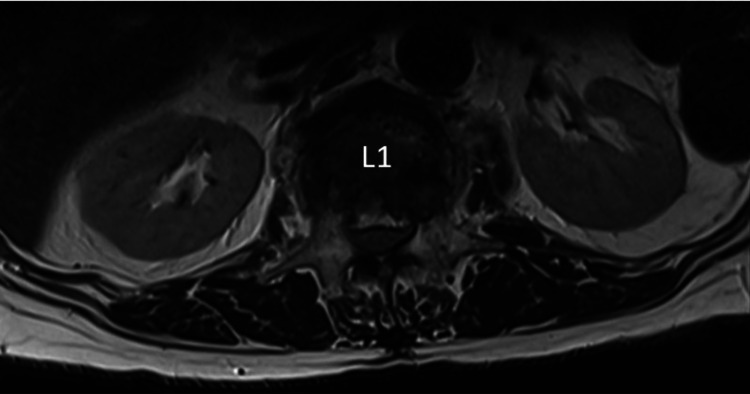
Preoperative T2-weighted MRI of the lumbar spine - axial view
